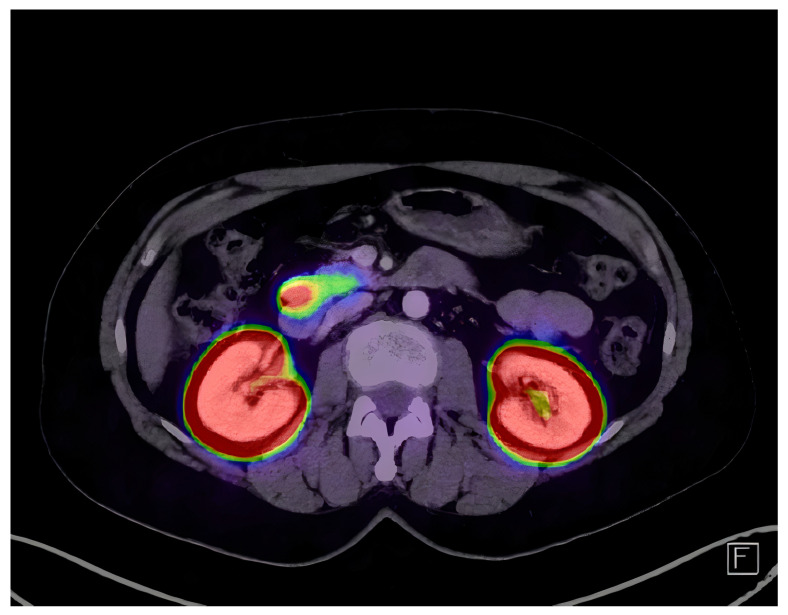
An axial PET/CT image shows a single intense 2 cm hyperperfused pancreatic lesion located anteriorly in the head of the pancreas. There is physiological tracer uptake in the kidneys. Exenedin-4 is a glucagon-like peptide 1 (GLP-1) receptor agonist that can image insulinomas that do not express somatostatin receptors in significant amounts for standard imaging.
